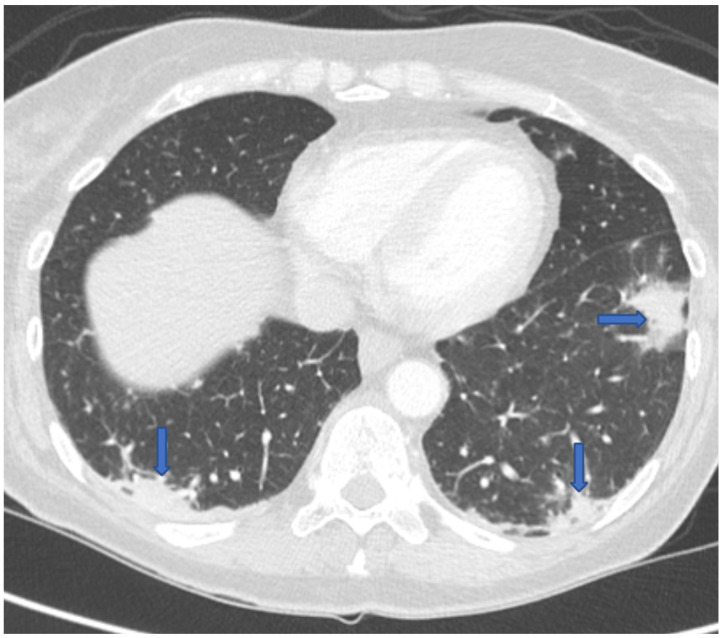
CT chest revealing multifocal bilateral peripheral opacities.
